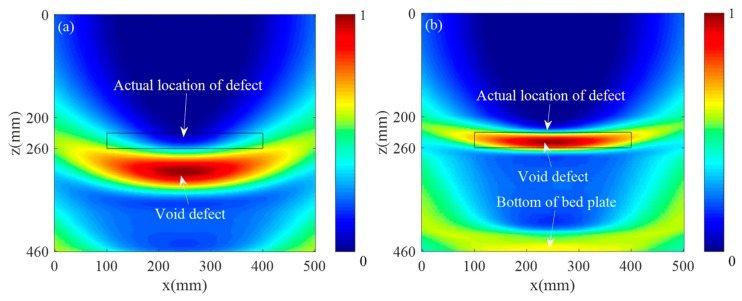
Ultrasound imaging results: (a) SAFT imaging and (b) multilayer SAFT imaging.
